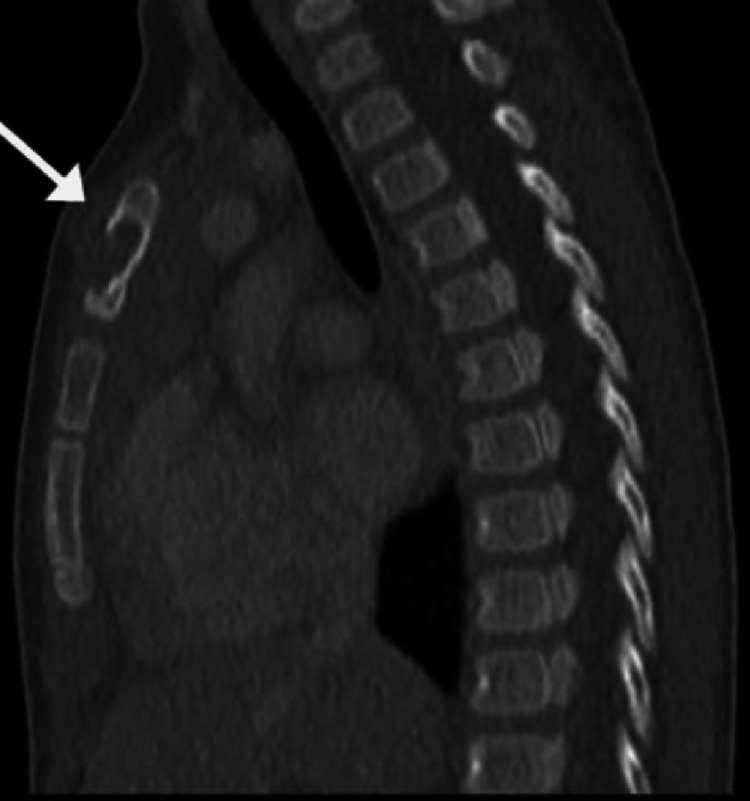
A 15-month-old girl with BCG osteomyelitis Sagittal reconstructed CT image shows a well-defined osteolytic lesion in the sternum with interruption of the anterior cortical continuity [17].
